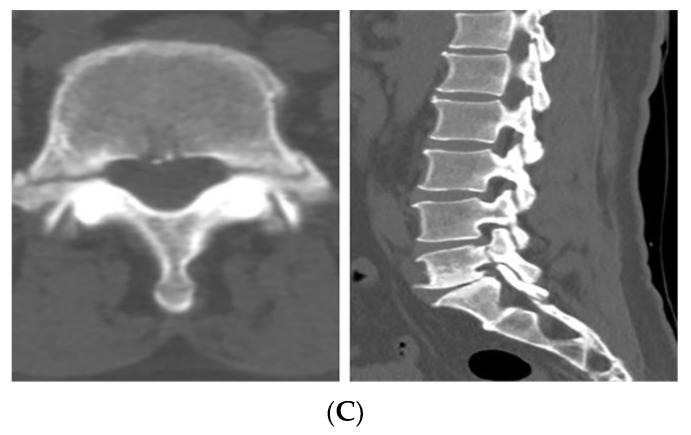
Initial radiologic evaluation at the first visit to our hospital. (A) The image shows a subtly visible fracture at the pedicle of L5 and Meyerding grade 1 spondylolisthesis at the L5–S1 level. (B) Flexion–extension lateral radiographs showing no relative sagittal plane translation between L5 and S1. (C) CT images confirming isolated bilateral pedicle fractures at L5 without additional fractures. No sclerotic change, pseudoarthrosis, or pars defect was observed, consistent with an acute fracture.
